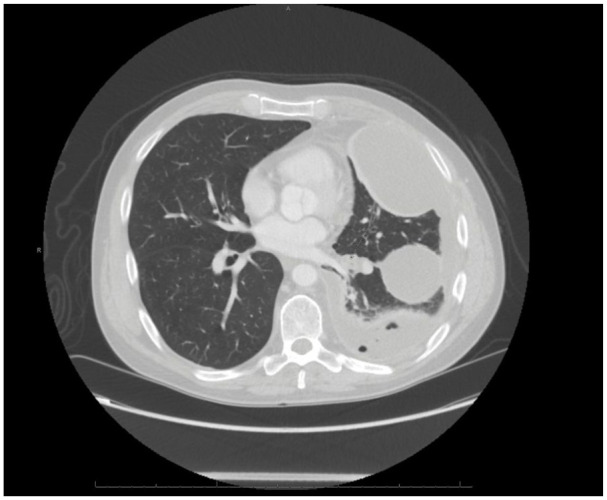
CT chest with contrast showing subtle filling of proximal left lower lobe bronchi without an obvious endobronchial lesion and left-sided empyema.
